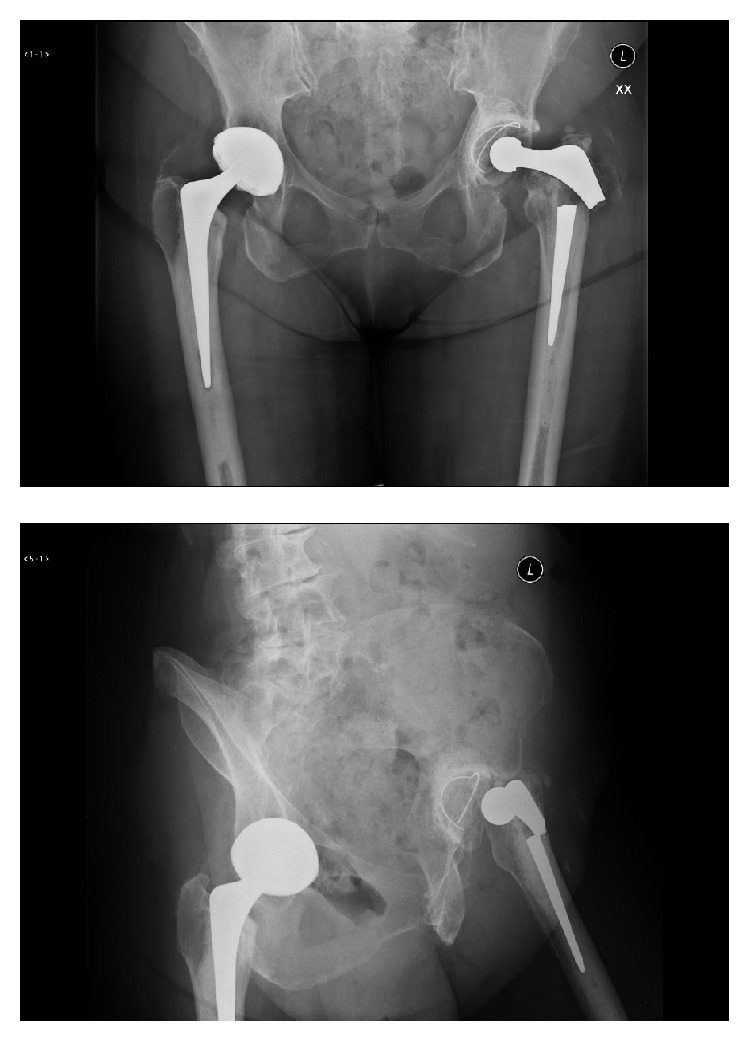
AP and Judet radiograph of the pelvis showing fracture through the left femoral stem prior to retrieval.
